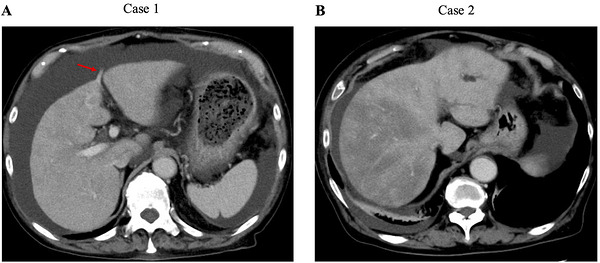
Radiological and pathological findings of the two cases. (A) Abdominal CT in Case 1 showed moderate to severe ascites and a patent umbilical vein (red arrow). (B) Abdominal CT in Case 2 showed mild ascites and a mosaic pattern of enhancement.
